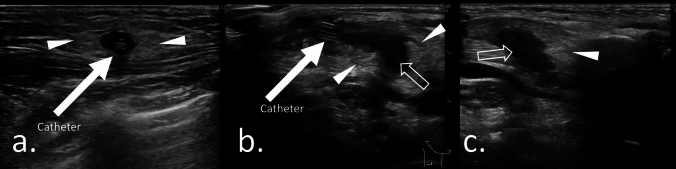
Case 8: Ultrasonographic findings in a 3.3-year-old boy with swelling, erythema, fever, and unplanned CVC extraction. The patient was diagnosed with complications within the subcutaneous tissue due to catheter infection at the tunneled area. The onset of symptoms was 296 days after catheter placement. The pathogen was *Staphylococcus aureus*. Catheter removal was performed 1 day after symptom onset. a. Ultrasonography showing a hyperechoic area (solid arrowheads) around the catheter. b. Ultrasonography indicated the presence of fluid collection (vacant arrow) around the catheter accompanying hyperechoic subcutaneous tissue (solid arrowheads). c. Ultrasonography showing fluid collection around the catheter (vacant arrow) without hyperechoic foci. A hyperechoic area is evident (solid arrowhead) within subcutaneous tissue
